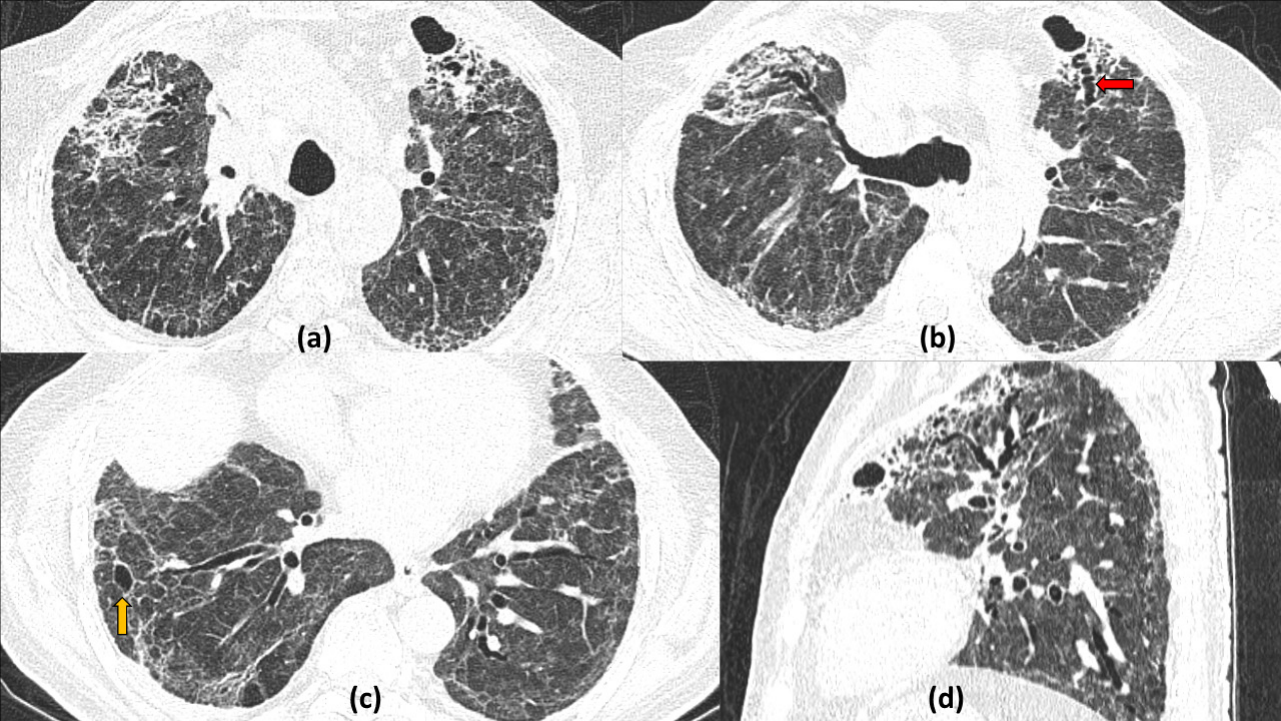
Cystic bronchiectasis and air spaces in sequelae of COVID19 infection: A 61-year-old male with severe COVID19 infection 2 months back, now RTPCR negative with recurrent dyspnoea. Axial (a–c) and sagittal reformatted (d) Chest HRCT sections show bilateral global tubular bronchiectatic changes. An addition few small cystic lesions are seen in the anterior paraseptal region of left upper lobe which are in continuation with a dilated bronchus (red arrow in b) suggestive of cystic bronchiectasis. Another cystic air space is also seen in the lateral basal segment of right lower lobe (yellow arrow in c). Note is made of marked peripheral subpleural interlobular septal thickening, parenchymal bands, architectural distortion suggestive of post-COVID19 fibrotic changes.
